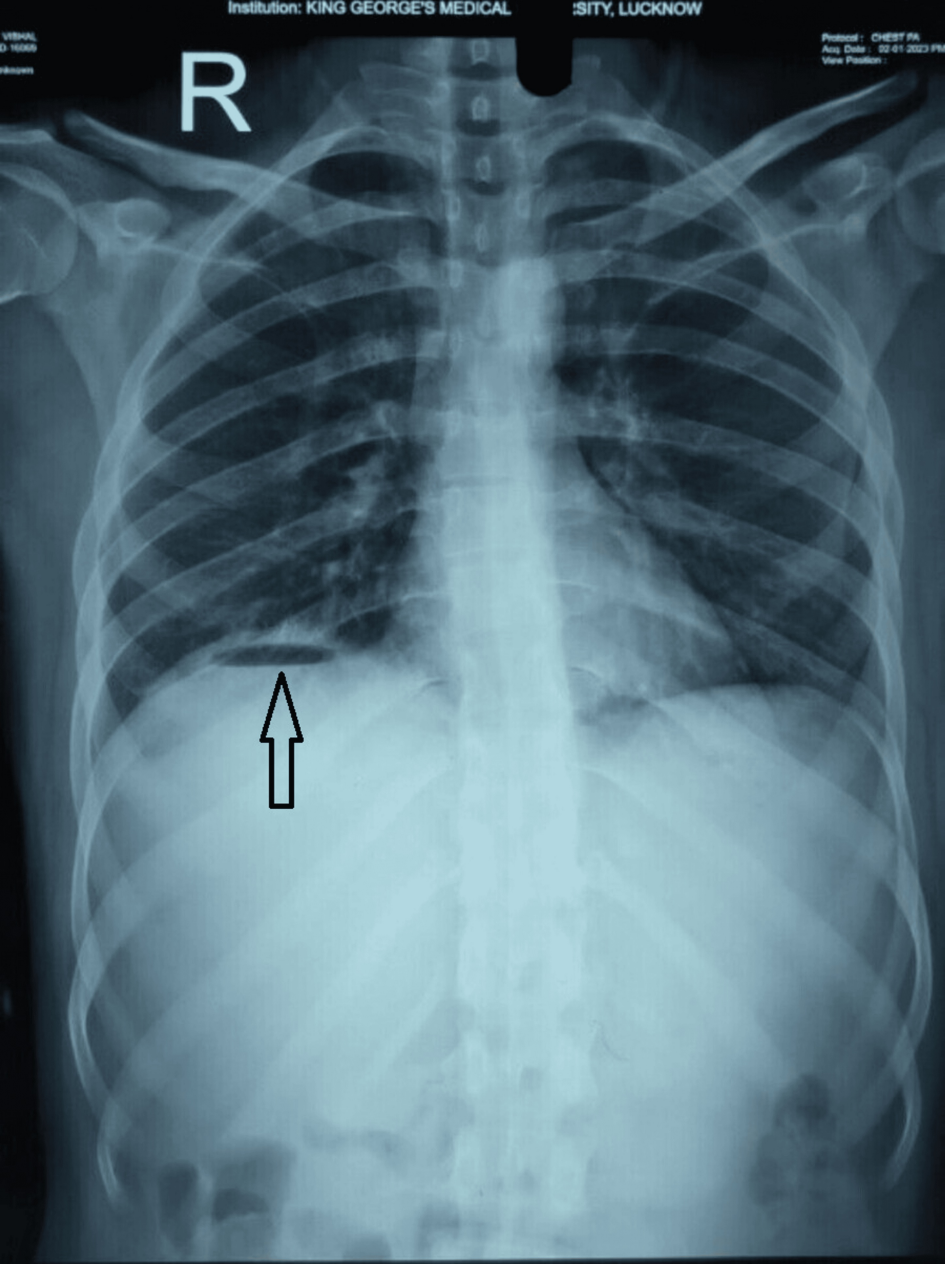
Abdominal X-ray showing gas under the right diaphragm (arrow)
